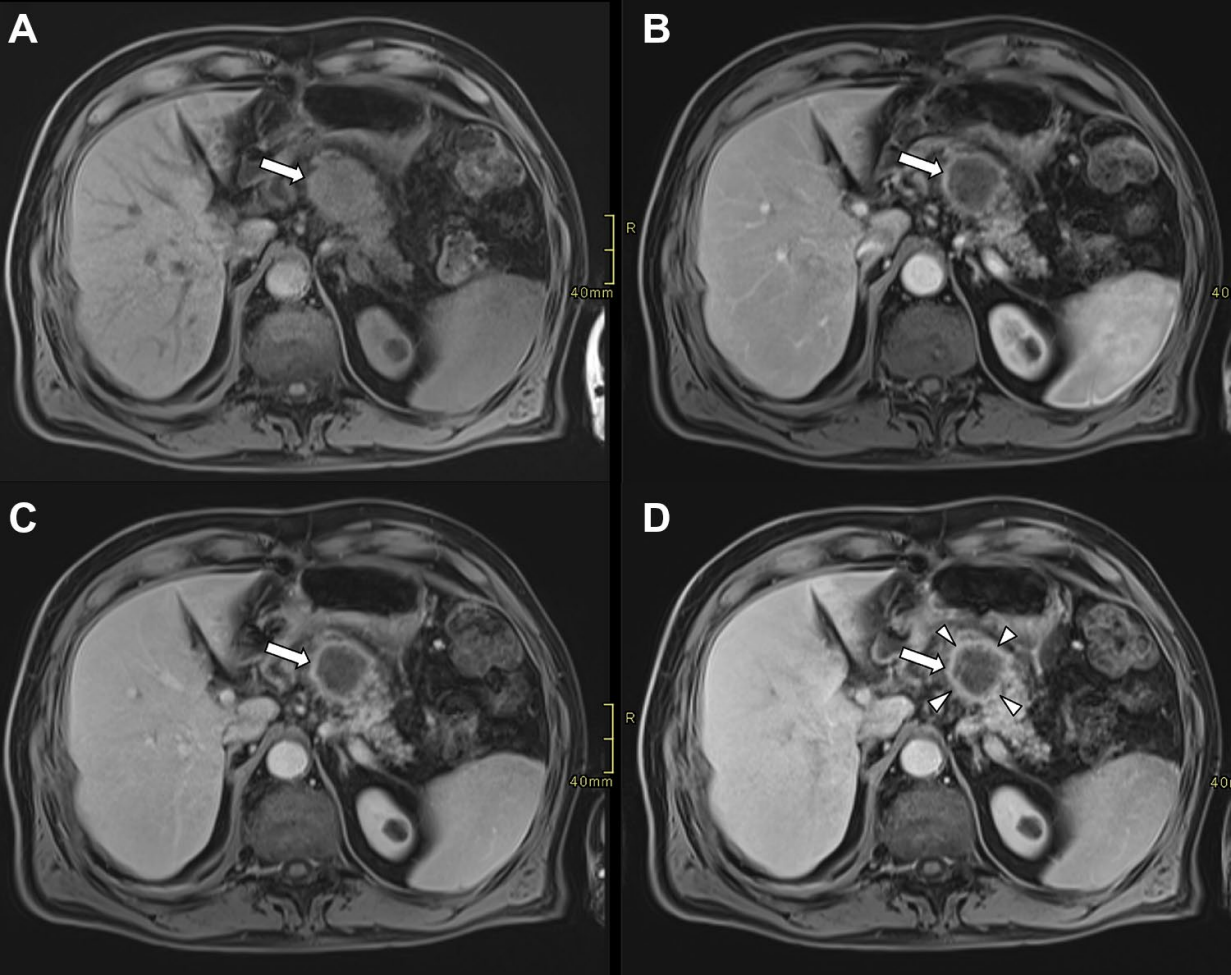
Axial contrast-enhanced MR images of a large pancreatic ductal adenocarcinoma at the pancreatic body (arrows). The tumor demonstrates hypointensity on T1-weighted (A) images. The lesion appears hypointense on pancreatic (B), portal venous (C) and delayed phases (D). In the delayed phase (D), a well-defined enhanced rim (arrowheads) becomes visible, delineating the lesion margin more clearly than in the earlier phases
